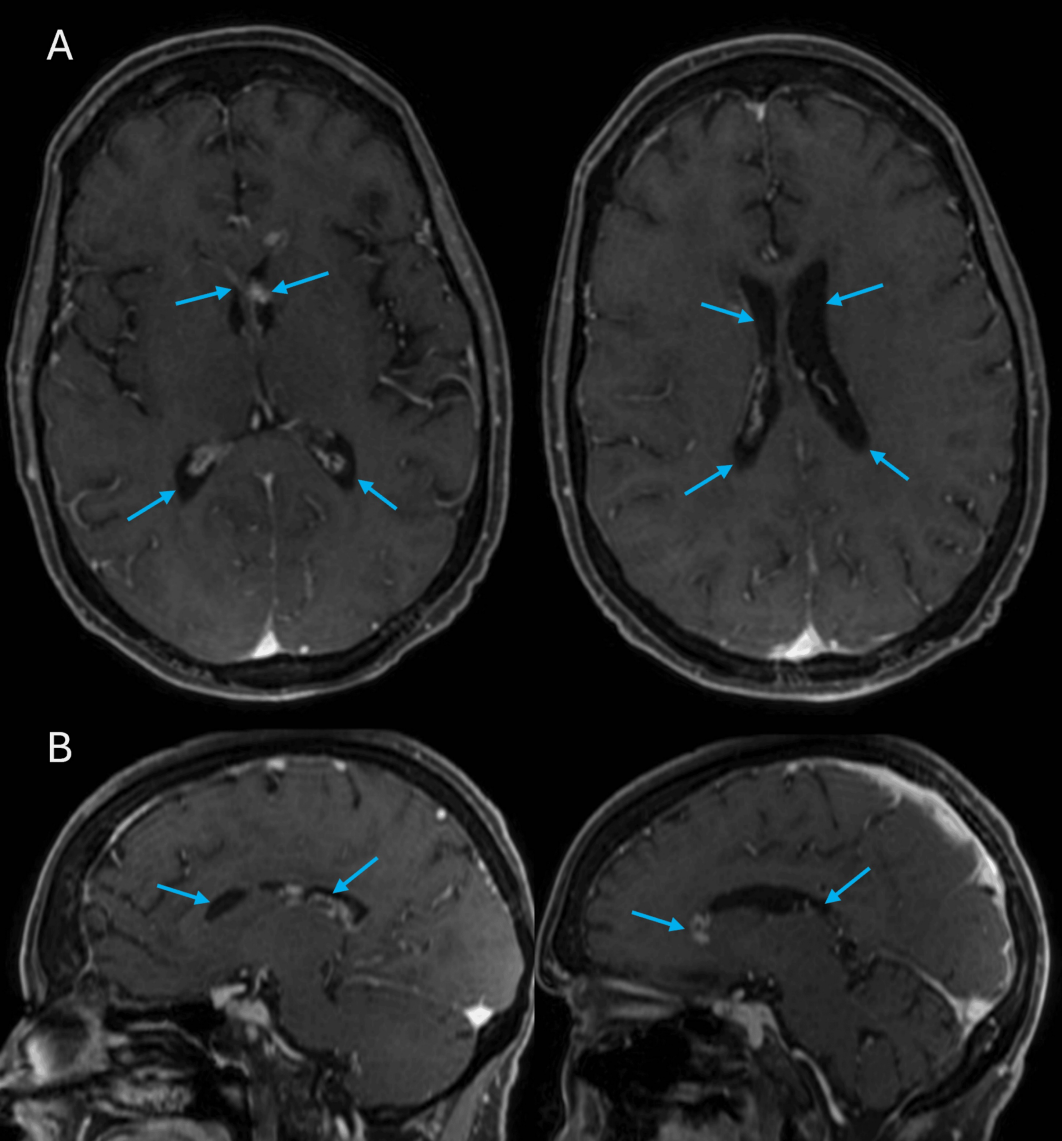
MRI scans showing considerable regression of the ventricular disease burden (A) Axial and (B) Sagittal T1 MRI scans with contrast showing substantial decrease in the size of the ependymal lesions of the lateral ventricles bilaterally (blue arrows)
